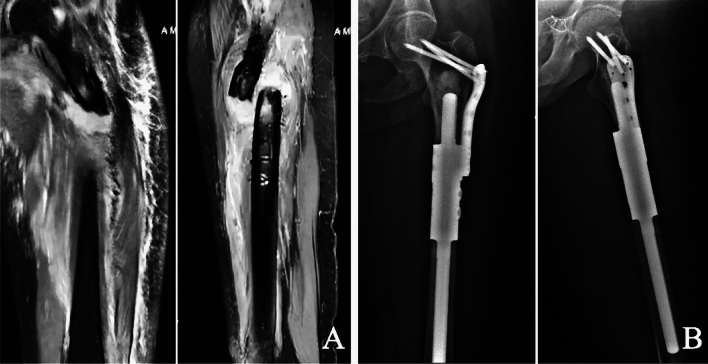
Cemented fixation of proximal femoral prosthesis in the 51-year-old woman above following resection of a metastatic carcinoma. (A) Fat-suppressed T2-weighted (T2W) images showing a pathological diaphyseal fracture which had intramedullary hematoma with fat necrosis and peripheral hematoma. (B) Anteroposterior and lateral radiographs showing that screws of the auxiliary lock plate were fixed in the femoral neck, and all screws were in the appropriate position.
